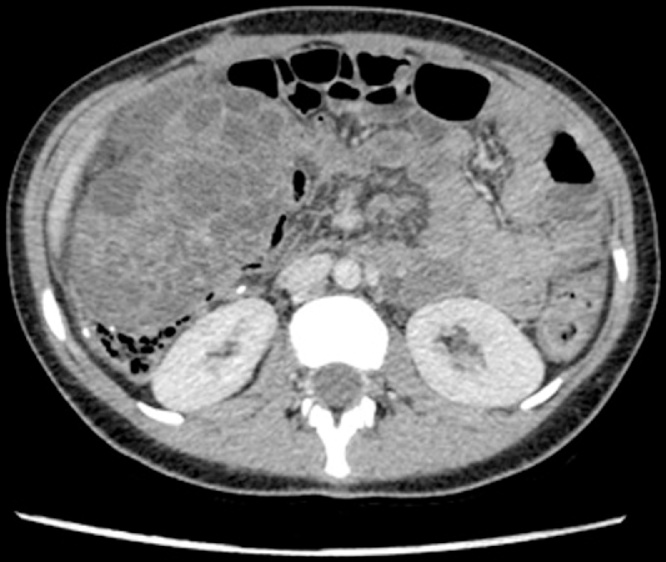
Follow up CT-SCAN of the abdomen that highlight the presence of two bulky pelvic mass both growing from ovaries.
